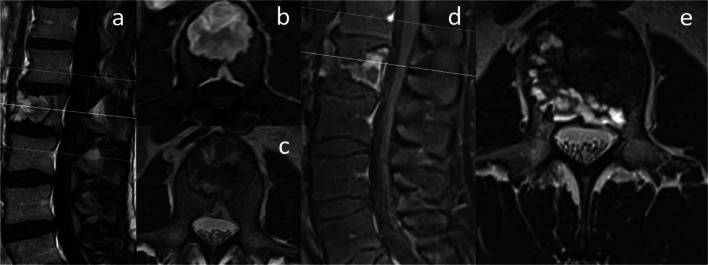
a, b Preoperative sagittal and axial T1-weighted and contrast-enhanced MRI dated 2016 revealing the osteolytic mass with medullary expansion, ground-glass matrix, narrow zone of transition, and a marginal sclerosis of L2, mainly affecting the anterior column. c Preoperative axial T2-weighted MRI dated 2016. d, e Preoperative sagittal and axial T1-weighted and contrast-enhanced MRI dated 2018 showing a progression of the osteolytic mass with a compression fracture of the upper endplate with increasing central cover plate recess at the posterior margin, affecting the anterior and middle spinal column leading to instability. No compression of nervous structures was observed
